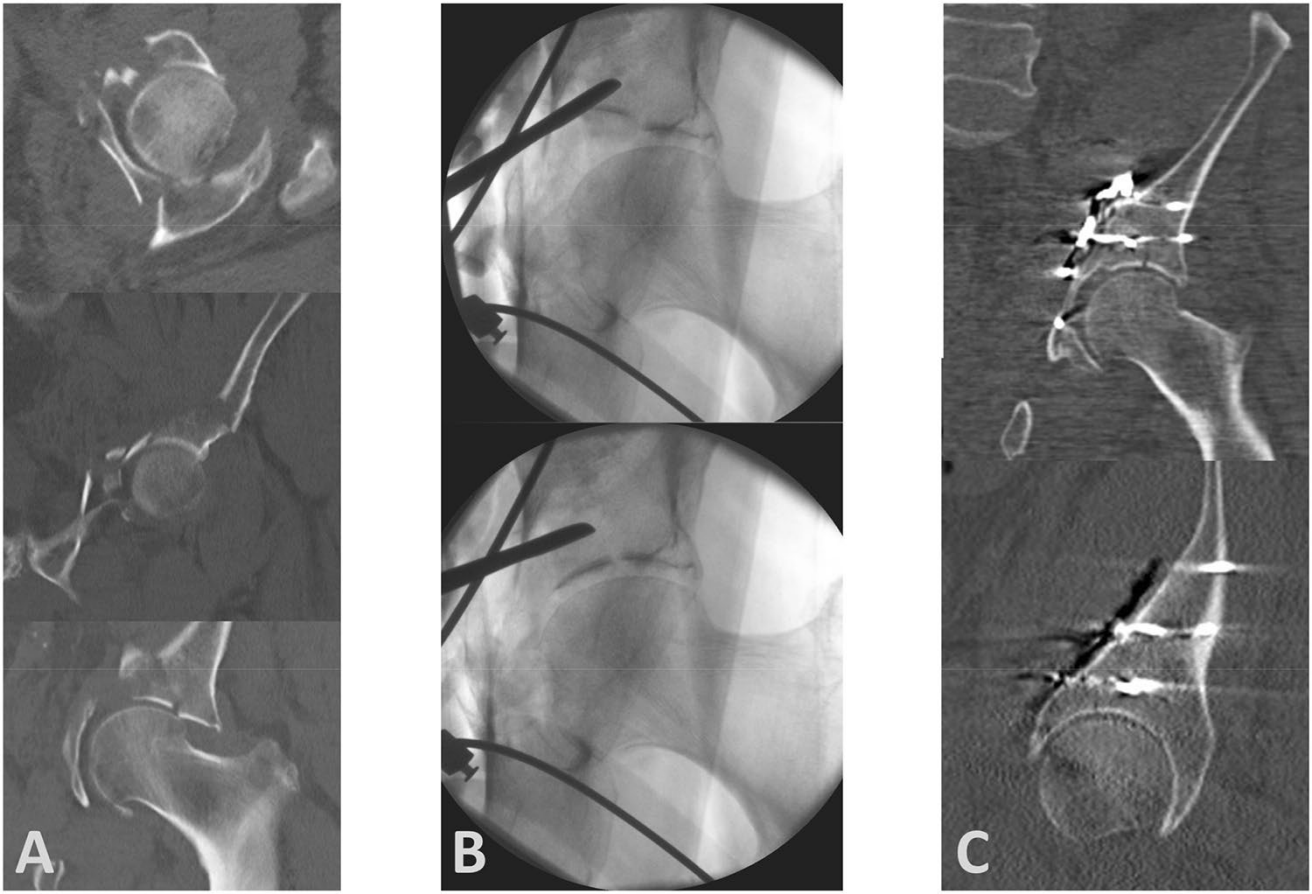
Example of an anterior column type acetabular fracture in a 72-year-old male with impaired bone quality and a large marginal impaction (gull sign). a Preoperative CT scan displaying a comminuted and impacted joint. b Intraoperative fluoroscopic images showing the head reduced from its subluxation using axial traction (above) and a reduction maneuver of the impacted joint surface using a rasp elevator. c Postoperative CT with a very successful yet imperfect reduction result (gap: 2 mm, step: 0 mm)
